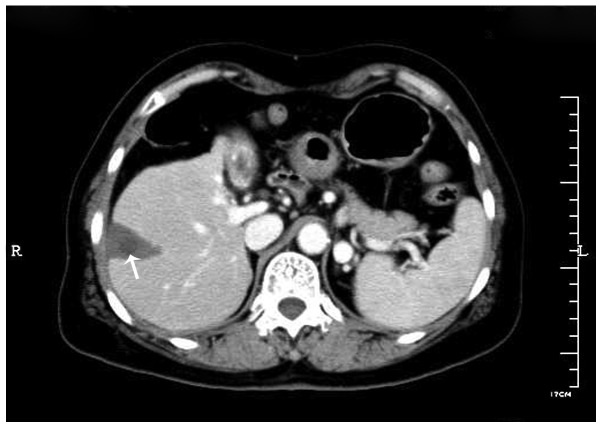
Abdominal CT image following hepatic RFA shows a wedge-shaped lesion of ablation extending to the outer edge of segment VIII in the liver. A low-density lesion 1.5 cm in diameter represents the location of the hepatic tumor, as indicated by the white arrow. CT, computed tomography; RFA, radiofrequency ablation.
